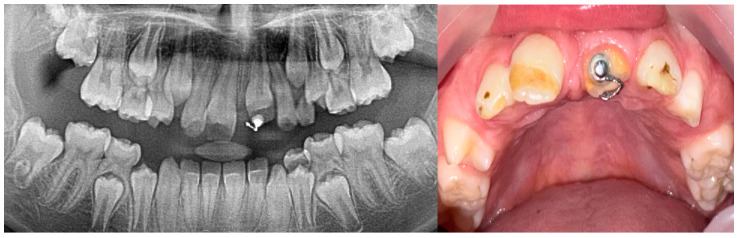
Radiographic findings and appearance of the erupted tooth in April 2025.
